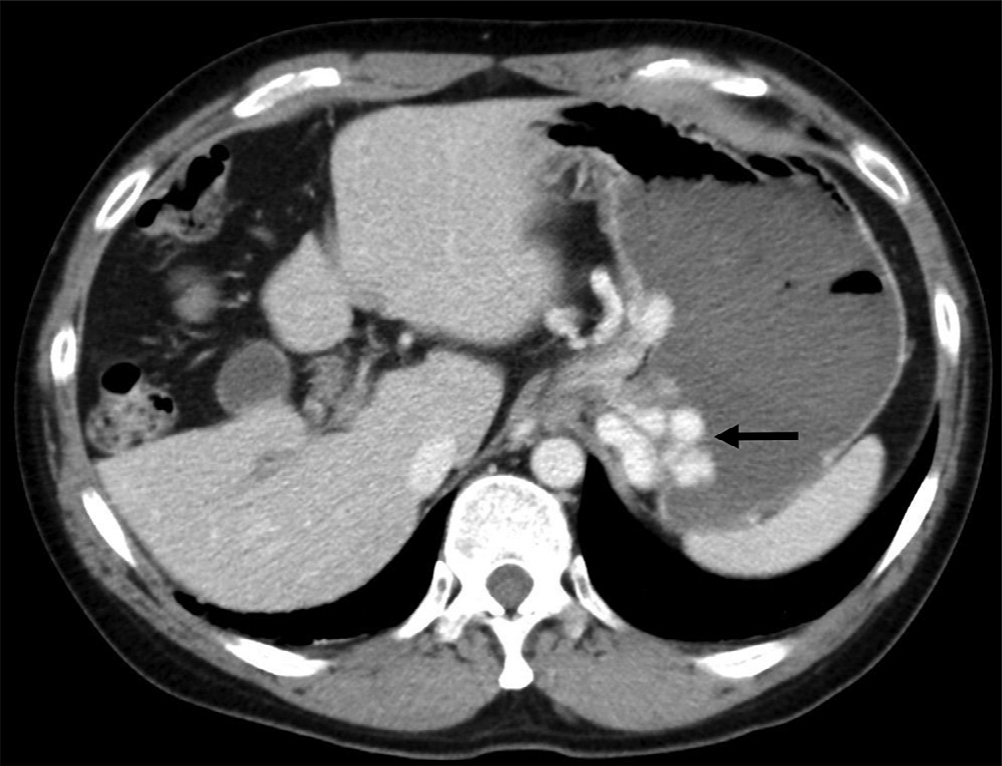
Contrast-enhanced CT before treatment shows large gastric varices (black arrow).
